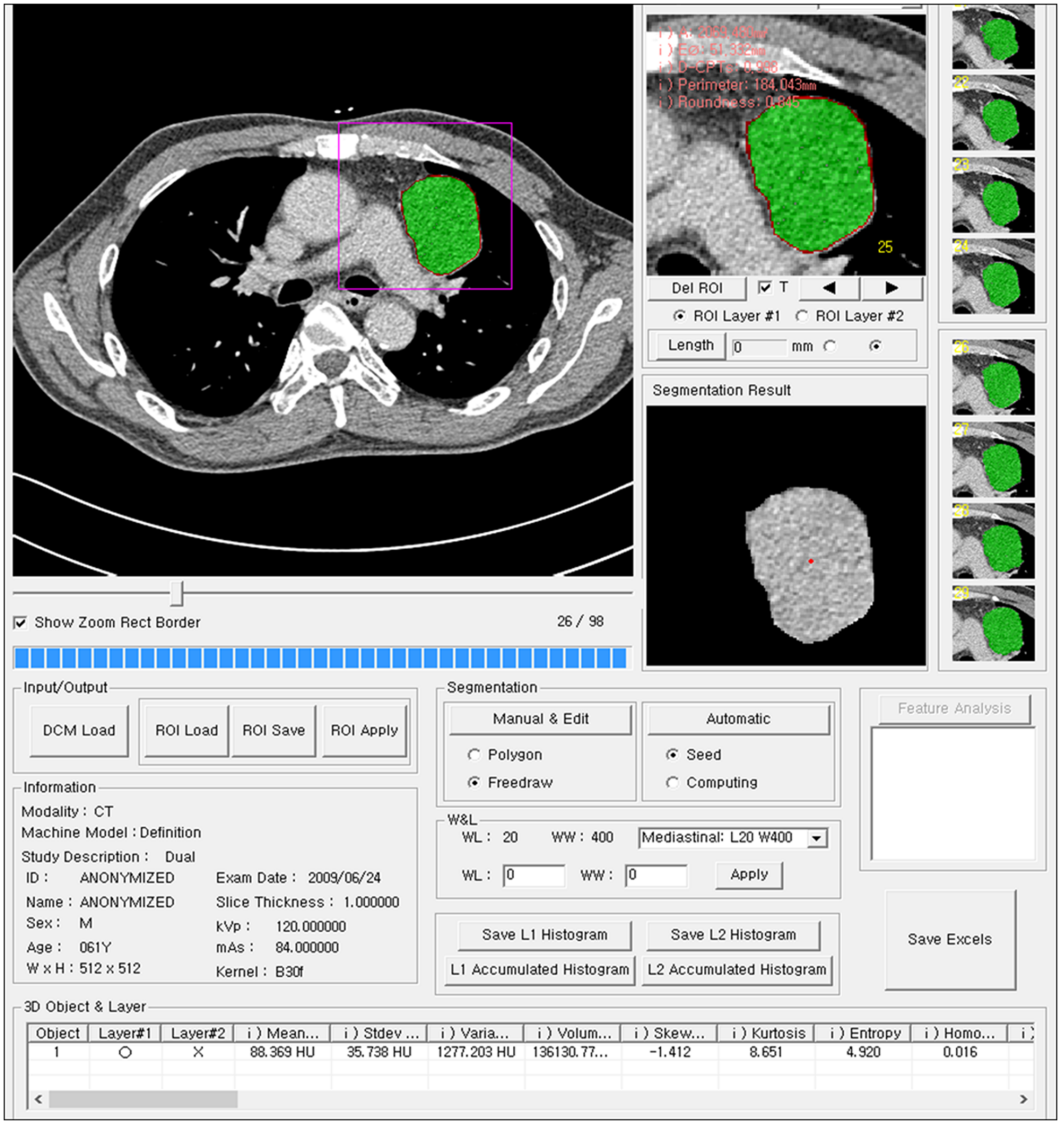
3D shape analysis software program. Each thymoma was manually segmented from surrounding structures on all CT images and their 3D shape features were automatically calculated using an in-house developed software program.
